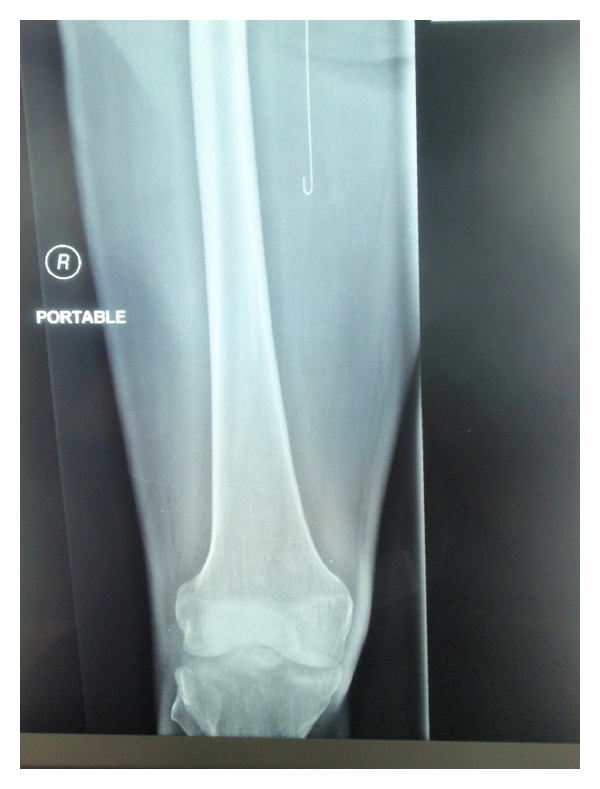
Right thigh X-ray showing the J-tip of the lost guidewire medial to the femur.
